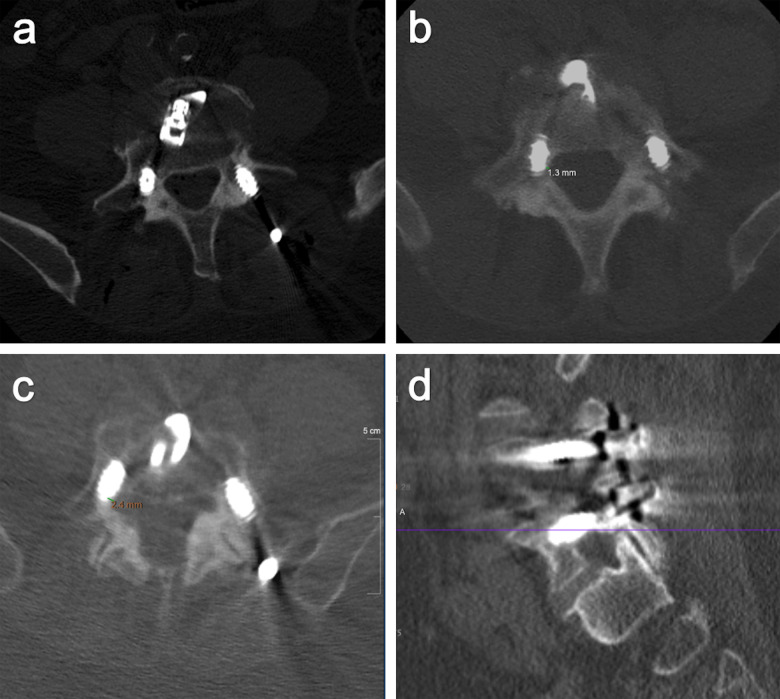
Postoperative computed tomography (CT) images of the L5 vertebral body of three separate patients demonstrating pedicle screw accuracy using the gertzbein and robbins classification system. Panel (a) demonstrates bilateral Grade A screw placement. Panel (b) shows right Grade B (<2 mm cortical breach) and left Grade A screw placement (no cortical breach). Panels (c) and (d) show a patient with a medial and inferior breach of the right L5 pedicle screw, classified as Grade C (<4 mm cortical breach) on axial (c) and sagittal (d) views. No Grade D or E cases are shown as there were none observed in this study.
